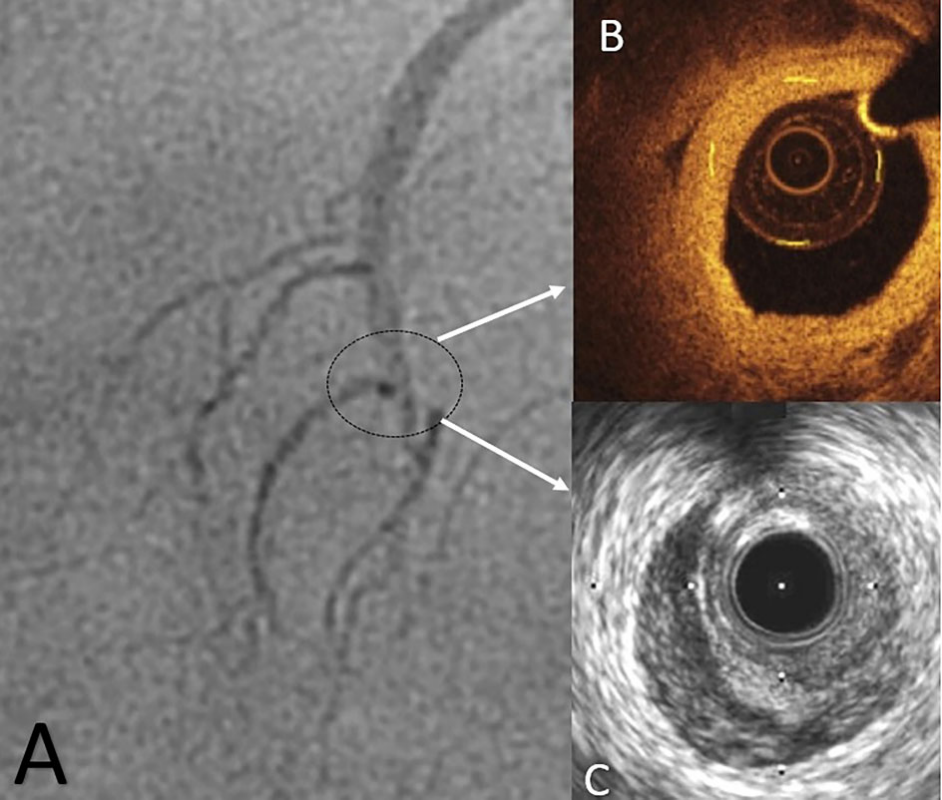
Pre-percutaneous coronary intervention images of a 52-year-old female. A, Clear coronary angiographic image. The black circle shows suspected coronary artery dissection. B, Frequency domain optical coherence tomographic image of the suspected part which clearly shows the lumen. Minimum lumen diameter: 2.08±0.09 mm, minimum lumen area: 3.25±0.01 mm^2^. C, Intravascular ultrasound image of the suspected part which clearly shows the lumen. Minimum lumen diameter: 2.18±0.1 mm, minimum lumen area: 3.48±0.01 mm^2^.
